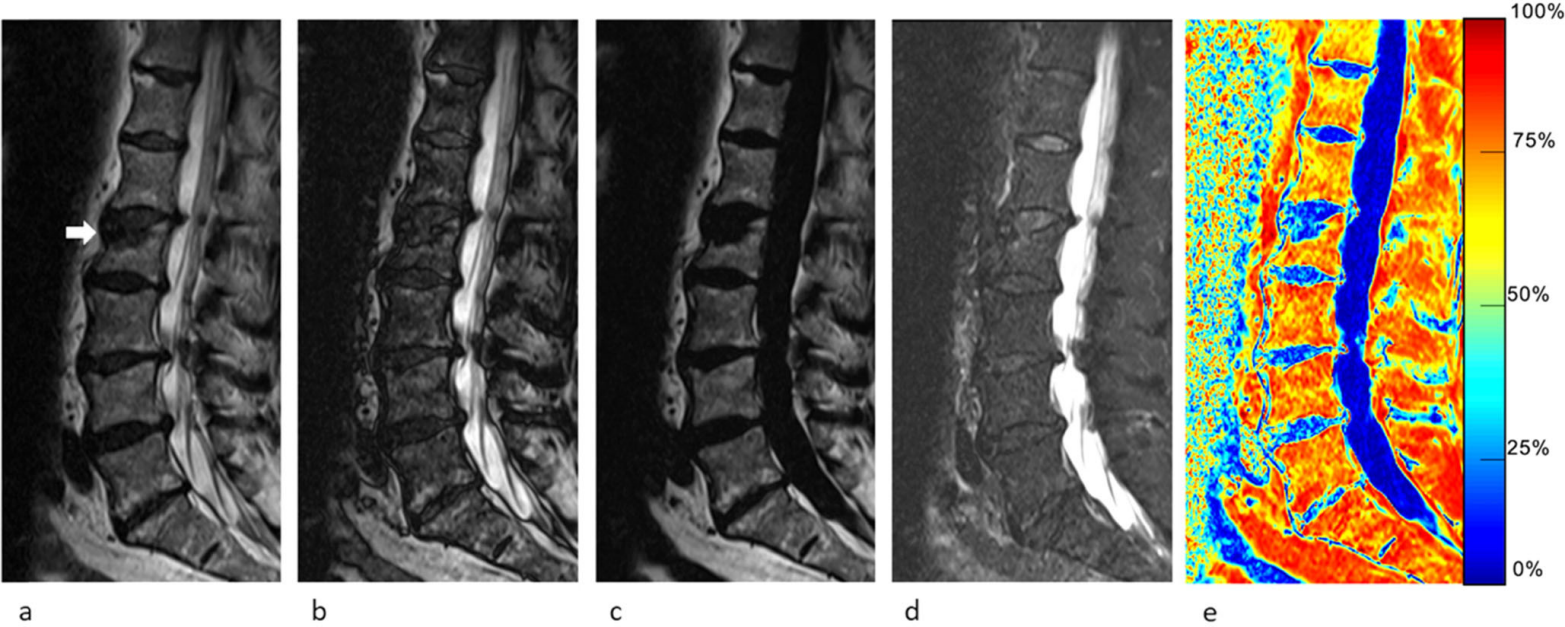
70-year-old woman with a benign vertebral compression fracture (VCF) at L2 level (arrow). Sagittal lumbar spine MR images generated from a signal FSE T2-weighted Dixon sequence include (a) in-phase, (b) out-of-phase, (c) fat-only, (d) water-only images, and (e) fat fraction (FF) map. At qualitative assessment, one reader wrongly qualified the VCF as malignant, but quantitative analysis correctly identified the benign nature of the VCF using the thresholds determined in this study (signal drop, FF calculated from water-only and fat-only images, and FF from maps were ROI1: 41.9%/ROI2: 40.4%; ROI1: 65.7%/ROI2: 32.3%; and ROI1: 73.9%/ROI2: 32.4%, respectively)
